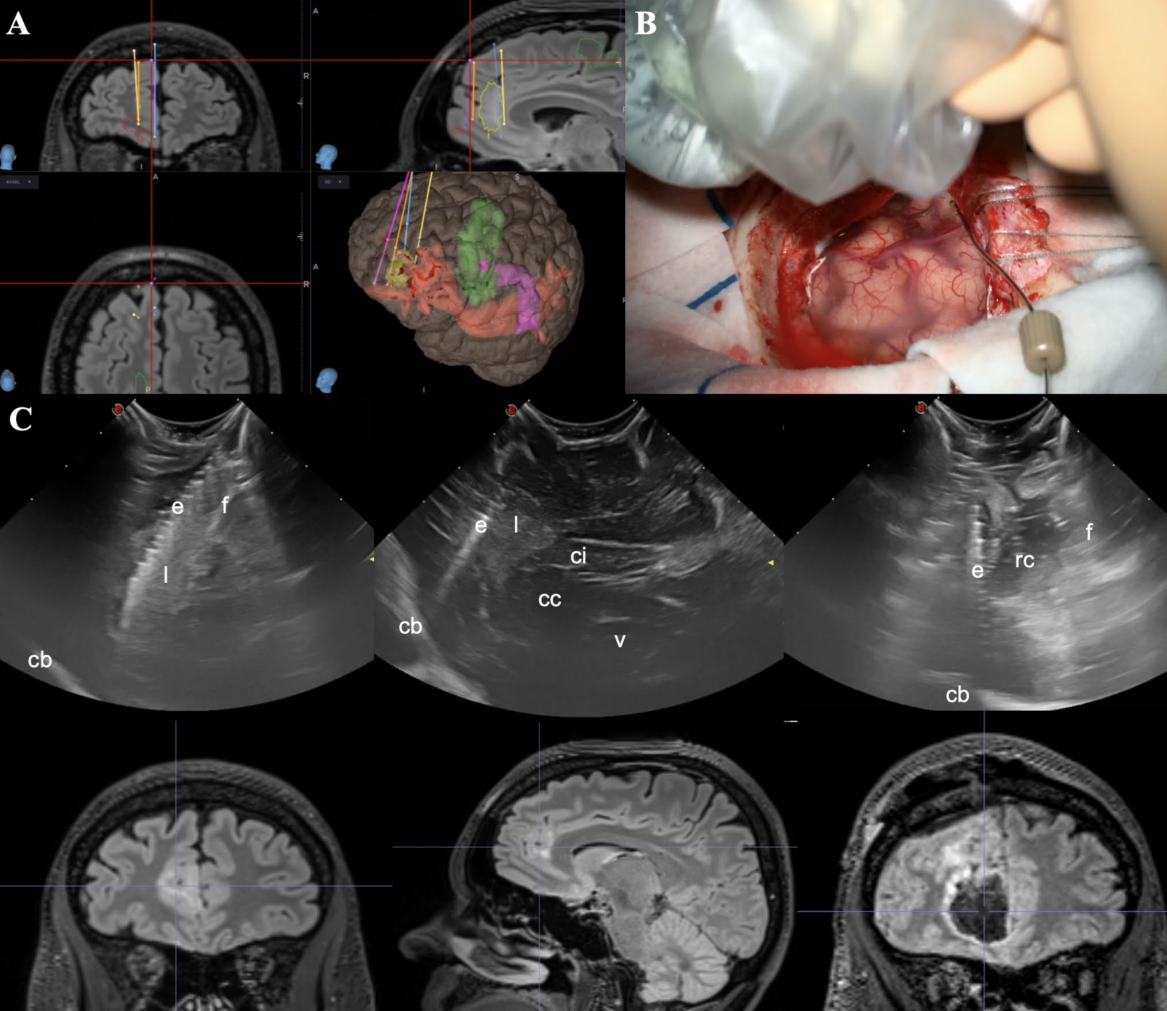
Presurgical neuronavigation planning and depth electrode positioning with ioUS. (Panel A) Preoperative neuronavigation planning, including 3D reconstructions of the lesion (yellow), major white matter tracts (frontal aslant tract, green; inferior fronto-occipital fasciculus, red; arcuate fasciculus, violet), and planned trajectories for intracerebral depth-electrode iECoG exploration. (Panel B) Intraoperative ioUS acquisition over the depth electrode positioned at the anteromesial border of the lesion. (Panel C) ioUS images compared with corresponding MRI cuts, flipped into anatomical orientation for better comprehension. Coronal and sagittal ioUS views show the electrode within the hyperechogenic lesion and its relationship with the falx cerebri and corpus callosum. The last coronal ioUS image, obtained after resection, depicts the electrode lateral to the resection cavity during post-resective iECoG sampling. Abbreviations: cb, cranial base; cc, corpus callosum; ci, cingulate gyrus; e, electrode; f, falx cerebri; l, lesion; rc, resection cavity; v, ventricle
